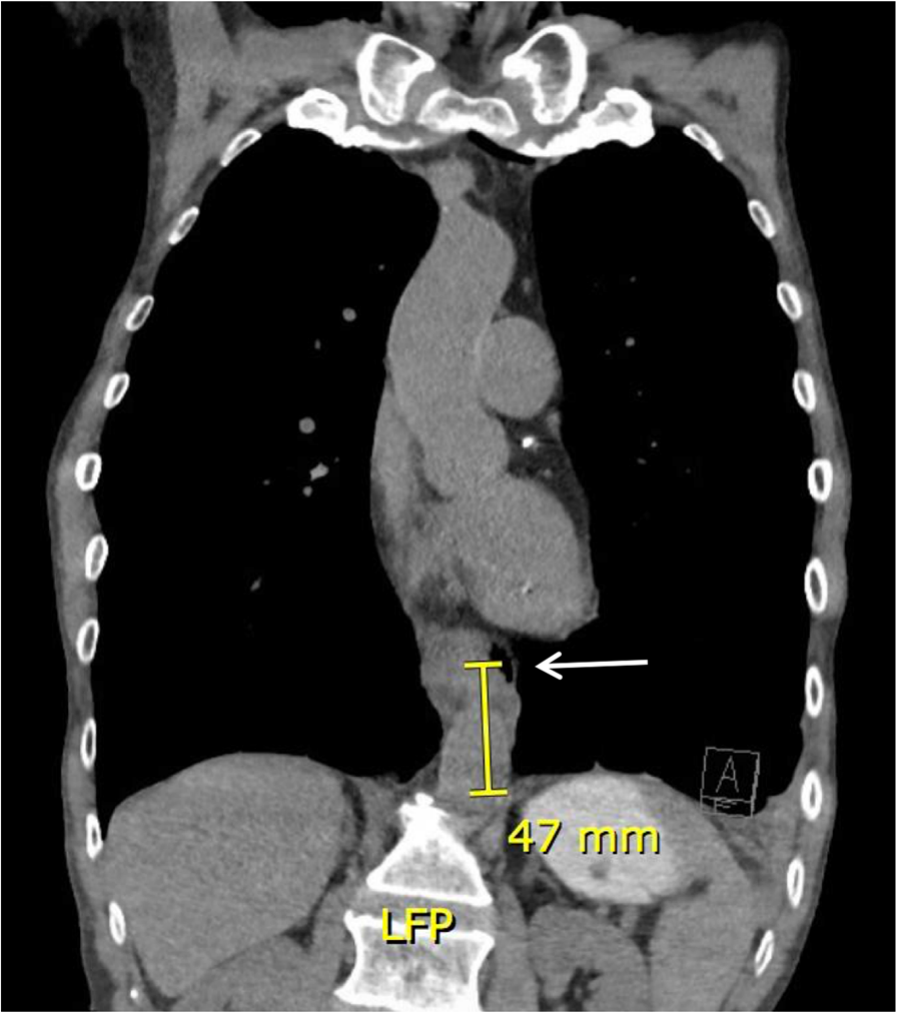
Computed tomography chest demonstrating an extramural gas locule adjacent to the left distal oesophagus about 47 mm from the GOJ (arrow), consistent with perforation. Coronal view. GOJ = gastro-oesophageal junction
